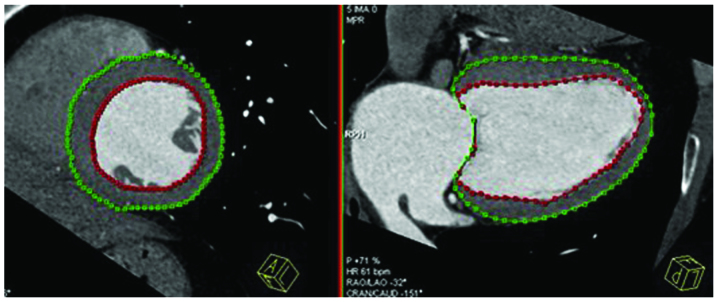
Example of the left ventricular systolic function calculated using short and long axis images from a dual source computed tomography coronary angiography.
